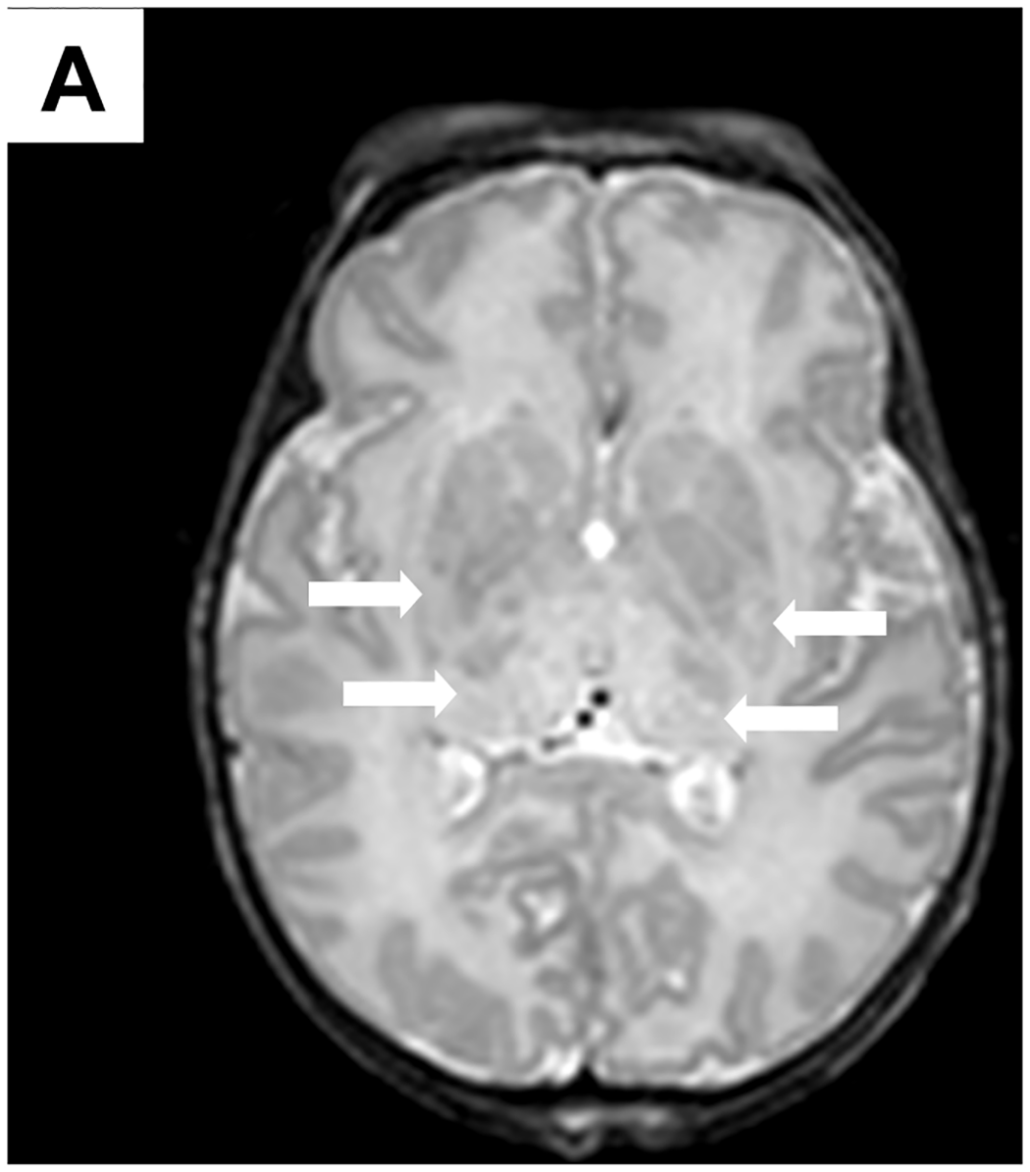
Brain MRIs of a term asphyxiated newborn treated with hypothermia, performed on day 9 of life. (A) The T2-weighted imaging shows the injury within the thalami and lentiform nuclei (arrows).
